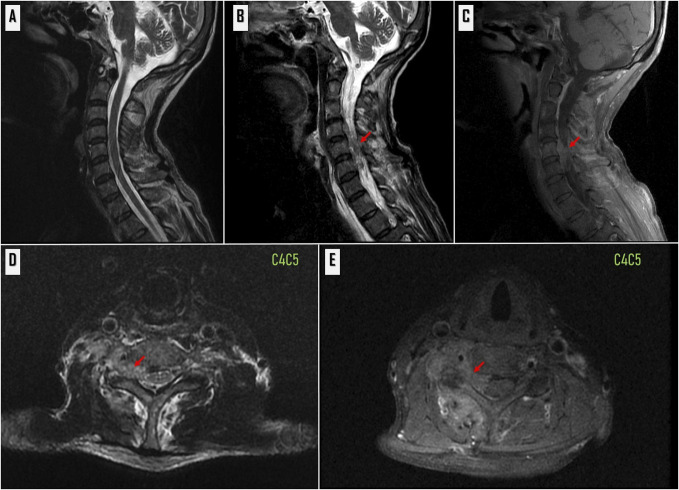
A, Mid‐sagittal T2‐weighted MRI shows no vertebral destruction. B, Right parasagittal T2‐weighted MRI and (C) T1‐weighted MRI withfat saturation and contrast demonstrate the located epidural mass posterior to the spinal cord and compression nerve root at the levelof C4‐C5(Red arrows). D, Axial T2‐weighted MRI and (E) T1-weighted with fat saturation and contrast MRI show the lesion located in the right posterior epidural space at the C4-C5 level.
